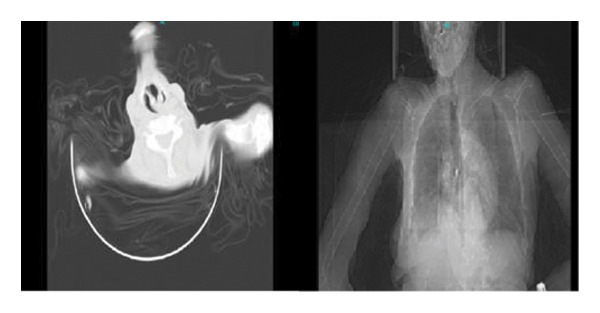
Chest high‐resolution computed tomography of a patient PCR‐positive for SARS‐CoV‐2 with cycle threshold 12 showing radiological features of atypical viral pneumonia CORADS 5 (reporting and data system CATEGORY 5) [4].
